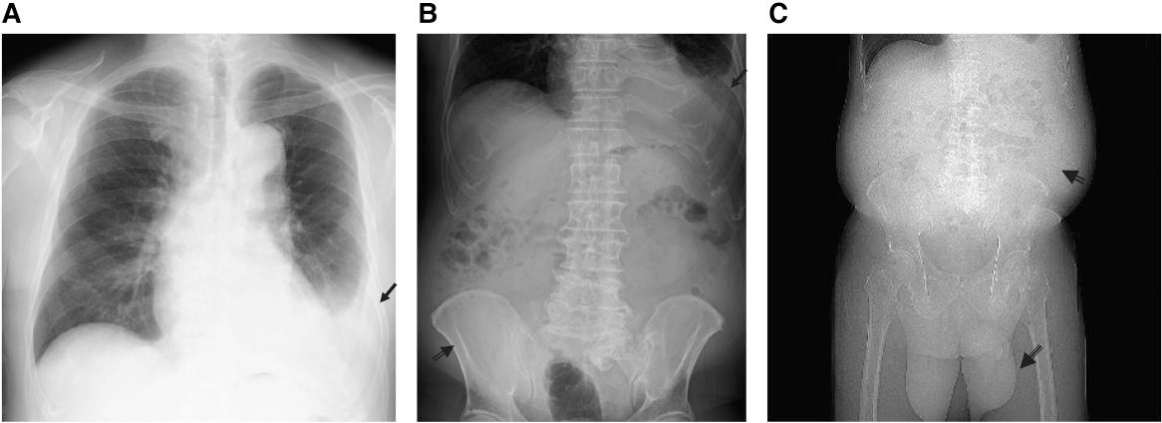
Pre-operative radiograph. (*A*) The single arrow indicates left pleural effusion. (*B*) The black double arrow indicates distended abdominal contents due to ascites. (*C*) The double arrows indicate the abdominal distended contents due to ascites, and the black triple arrow indicates the enlarged scrotum.
